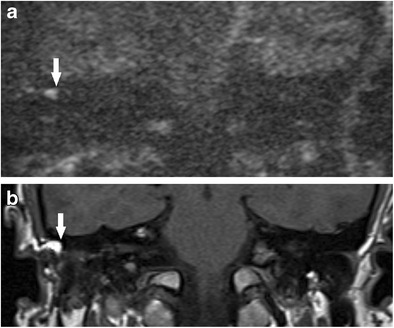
Value of non-contrast enhanced T1 weighted images. False positive high DWI signal (*white arrow*) for cholesteatoma was seen on a the b1000 image. b The non-contrast enhanced T1 weighted image showed this (*white arrow*) to represent subcutaneous tissue just outside the reconstructed post-operative middle ear cleft. Otoscopic examination of the area is normal and following discussion at the clinicoradiological meeting, a follow-up DWI was arranged. This showed stable appearance
